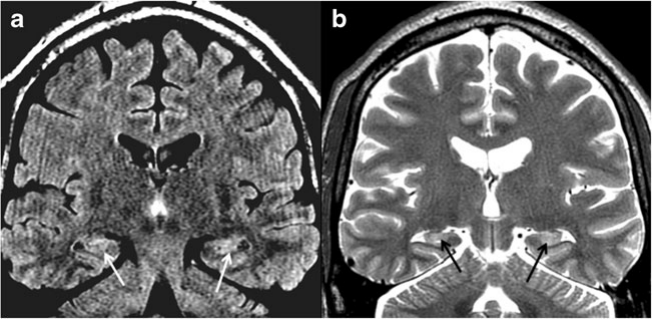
A 27-year-old female with persistent partial complex seizures. On coronal FLAIR (a) and T2 images, there is bilateral hippocampal atrophy and increased T2/FLAIR signal (arrows), compatible with bilateral mesial temporal sclerosis. Note mild diffuse atrophy possibly related to chronic epilepsy and drug effect
